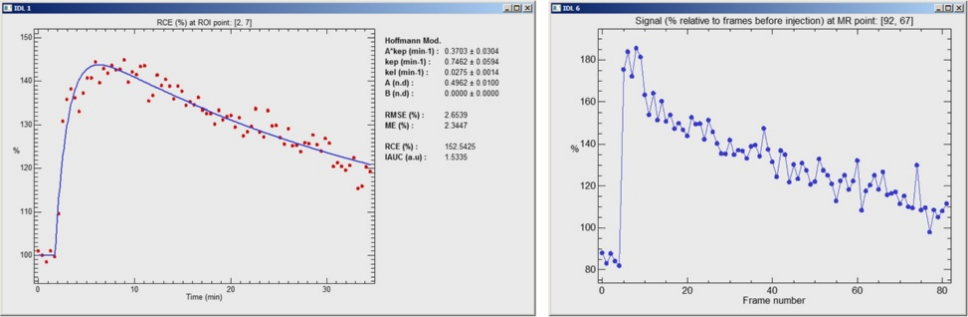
Modeled and acquired DCE-MRI curves. Modeled (left) and acquired (right) DCE-MRI curves. The software tool can plot the time-course changes in individual pixels or in the whole ROI. The curve can be compared with the analytic pharmacokinetic model (left plot), where the acquired data are represented as dots and the fitted evolution as a continuous curve.
